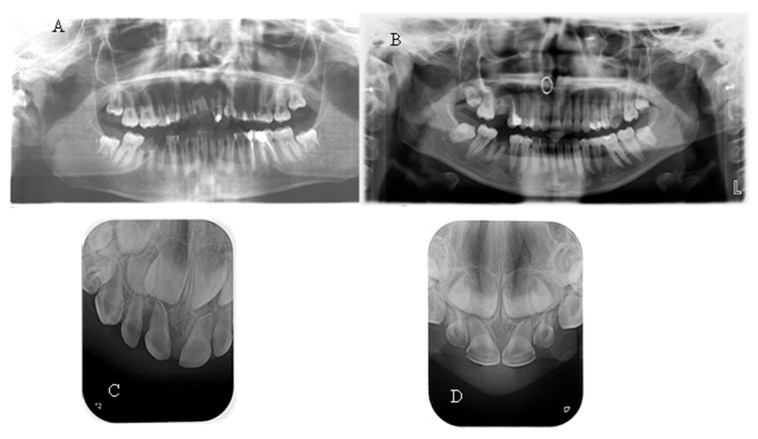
(A) OPG of a 15-year-old girl with XLH; (B) OPG of a healthy 15-year-old girl; (C) Periapical X-ray of a 3-year-old boy with XLH; (D) Periapical X-ray of a 3-year-old healthy boy.
